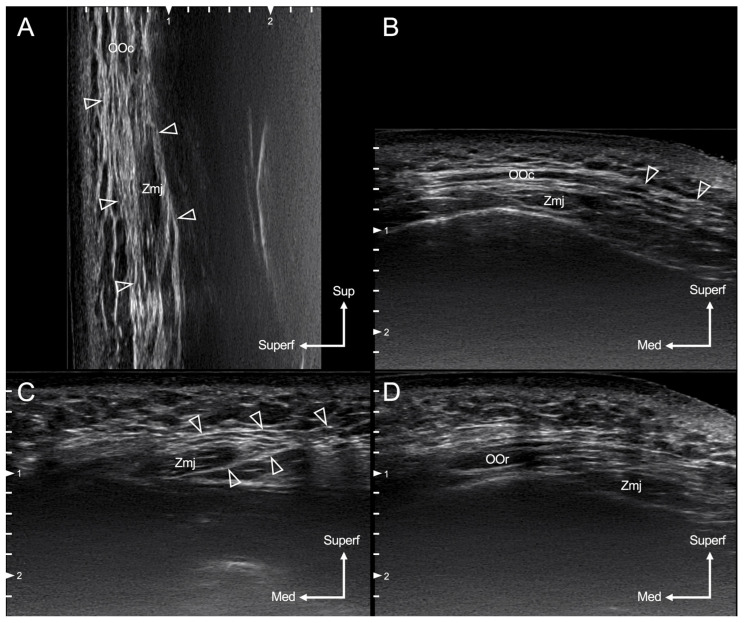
Ultrasound images of the layered relationships of the SMAS with the Zmj and OOc. The arising fibers of the Zmj at its origin were covered by the OOc and SMAS, with the SMAS extending (arrowheads) downward from the OOc, as observed in both longitudinal (A) and transverse (B) views. (C) Lateral to the vertical line passing through the lateral canthus, the Zmj fibers were enveloped by the SMAS both superficially and deeply (arrowheads), with the SMAS being thicker on the surface. (D) Prior to inserting into the orbicularis oris (OOr), the Zmj fibers were not clearly attached to the SMAS medial to the vertical line passing through the lateral canthus. Med, medial; Sup, superior; Superf, superficial.
